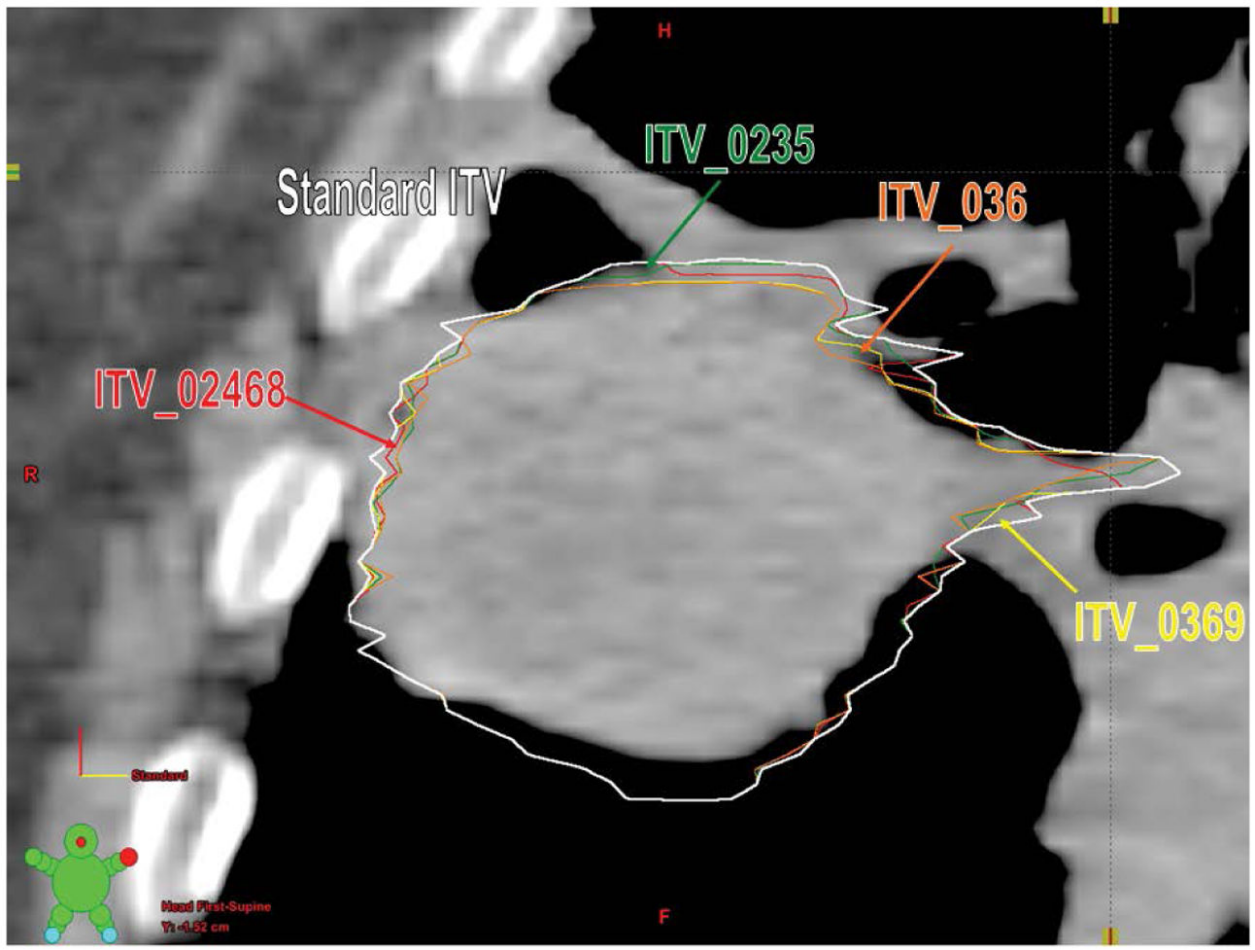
Transverse CT cut of a patient showing clinical ITV generated using all the 10 phases (Standard ITV). Also shown are the ITVs generated using a subset of all 10 phases represented as ITV_02468, ITV_0369, ITV_0235 and ITV_036 are the various samples which used (0%, 20%, 40%, 60, 80%), (0%, 30%, 60%, 90%), (0%, 20%, 30%, 50%) and (0%, 30%, 60%) to generate the respective ITVs.
